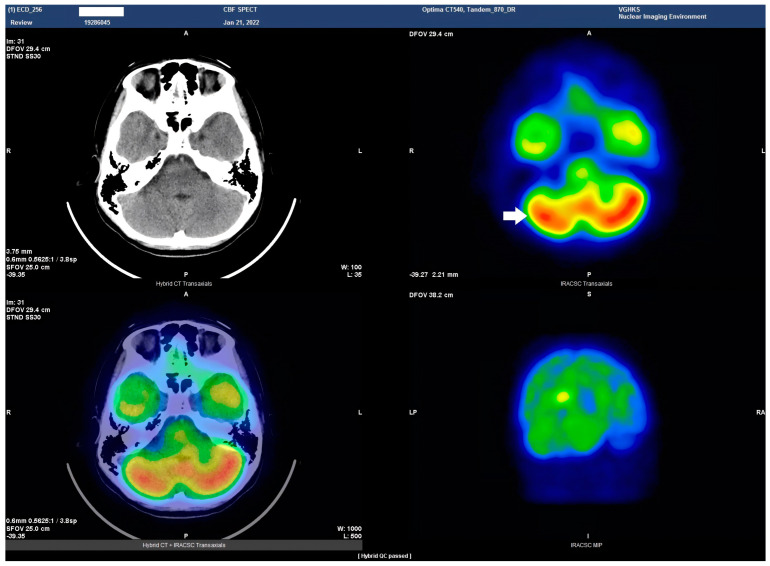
Axial view of the Tc−99m ECD brain perfusion SPECT study. A decreased uptake in the tracer(Tc−ECD) can be observed in the right cerebellar hemisphere (arrow). Left upper panel, CT image; right upper panel, SPECT; left lower panel, the combined SPECT and CT images.
